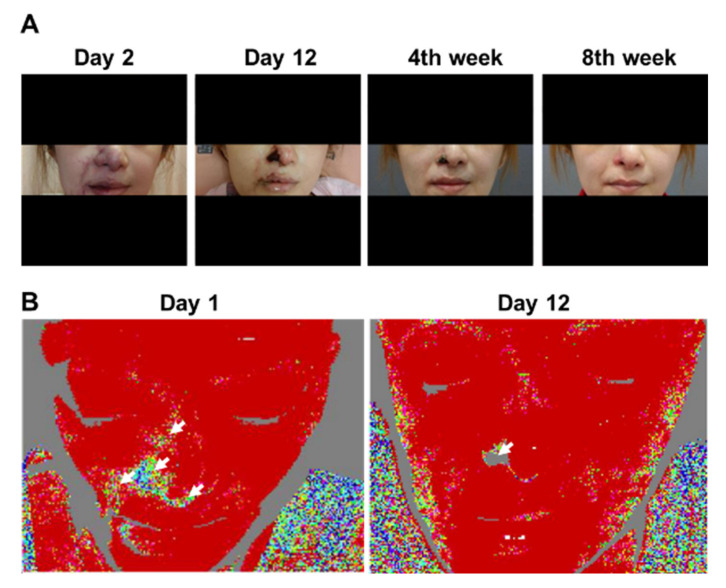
Clinical photographs and laser Doppler imaging of Patient 3. (A) Day 2 of admission; Day 12 of admission; Week 4 of follow-up; Week 8 of follow-up. Initial worsening and gradual improvement of cutaneous symptoms can be observed. Finally, only the right ala exhibited thick skin necrosis, which left an obvious scar. (B) Left panel: on Day 1 of admission, areas of cutaneous ischemia were clearly delineated from those without vascular occlusion. Right panel: on Day 12 of admission, although the originally affected area had not returned to its normal state of appearance, blood circulation of the other parts, except for the right ala, was restored.
